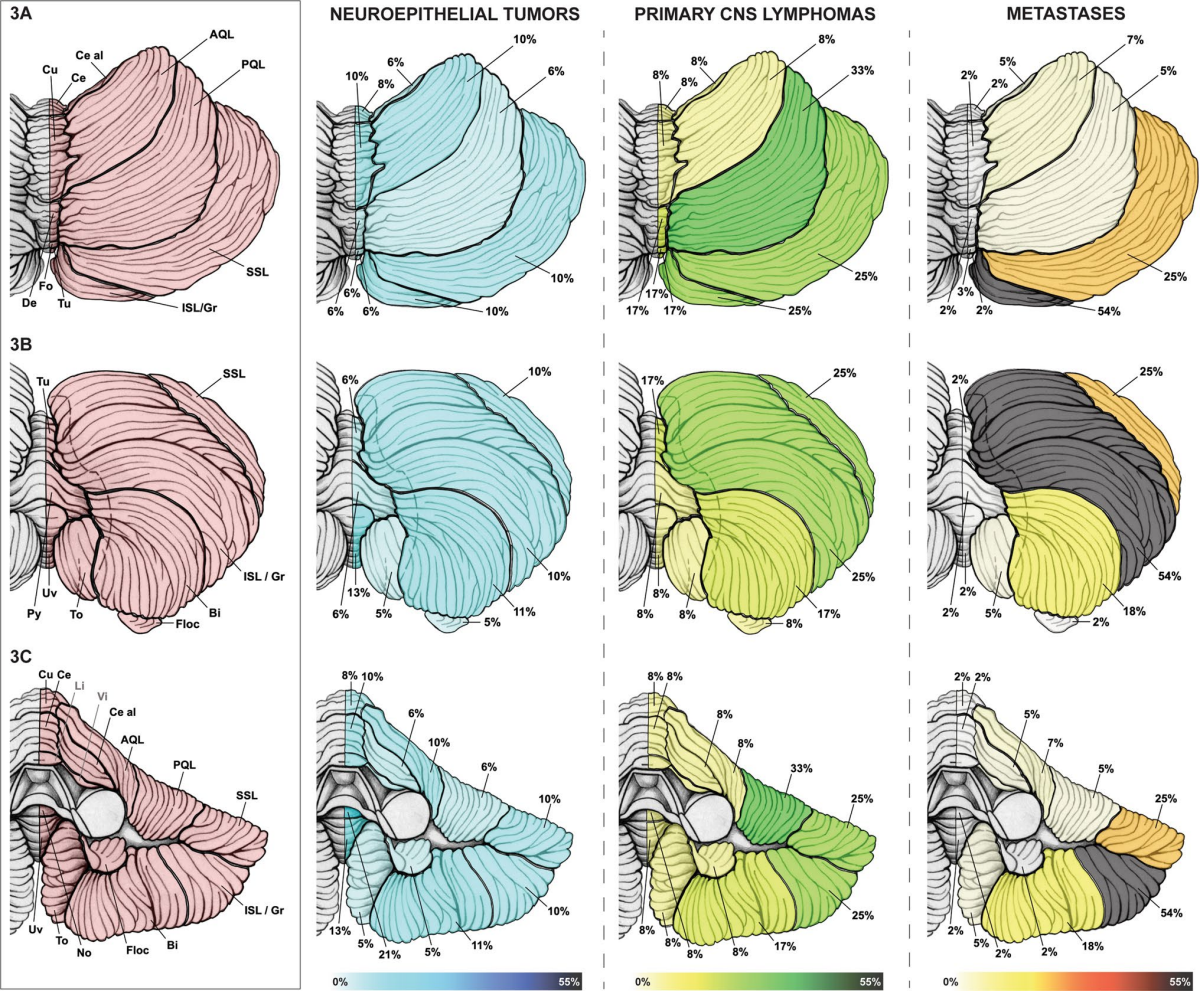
Surface topographic anatomy of cerebellar brain tumors: A superior, B inferior and C anterior view on the cerebellum with its vermian and corresponding hemispheric lobules. The cerebellar distribution of neuroepithelial tumors, primary CNS (central nervous system) lymphomas and metastases is shown. *AQL* anterior quadrangular lobule, *Bi* biventer/biventral lobule, *Ce* central lobule, *Ce al* ala lobuli centralis/wing of the central lobule, *CNS* central nervous system, *Cu* culmen, *De* declive, *Floc* flocculus, *Fo* folium, *ISL/Gr* inferior semilunar and gracile lobules—not separable on magnetic resonance imaging, *Li* lingual—not reliably separable on MRI, *No* nodule, *PQL* posterior quadrangular/simplex lobule, *Py* pyramid, *SSL* superior semilunar lobule, *To* tonsil, *Tu* tuber, *Uv* uvula, *Vin* vinculum—not reliably separable on MRI
